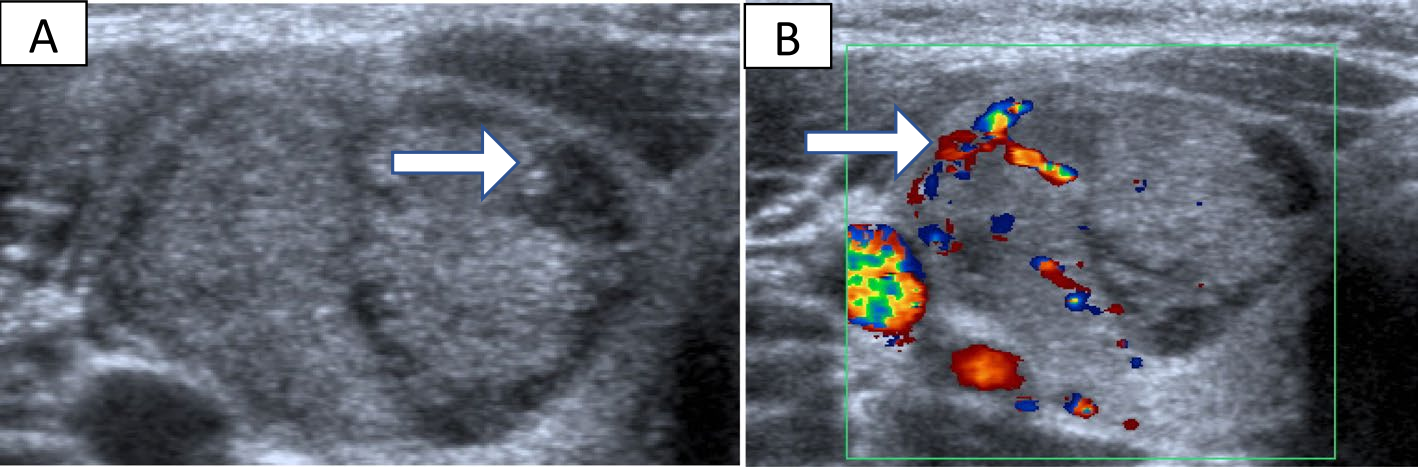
Thyroid ultrasound for patient 6; a 20 year old female who presented with a neck lump. Thyroid ultrasonography showing a 3 cm × 2.2 cm heterogenous thyroid nodule with focal areas of hypoechogenicity (arrow) (3A) and areas of vascularity (arrow) within the nodule (3B) (U3)
